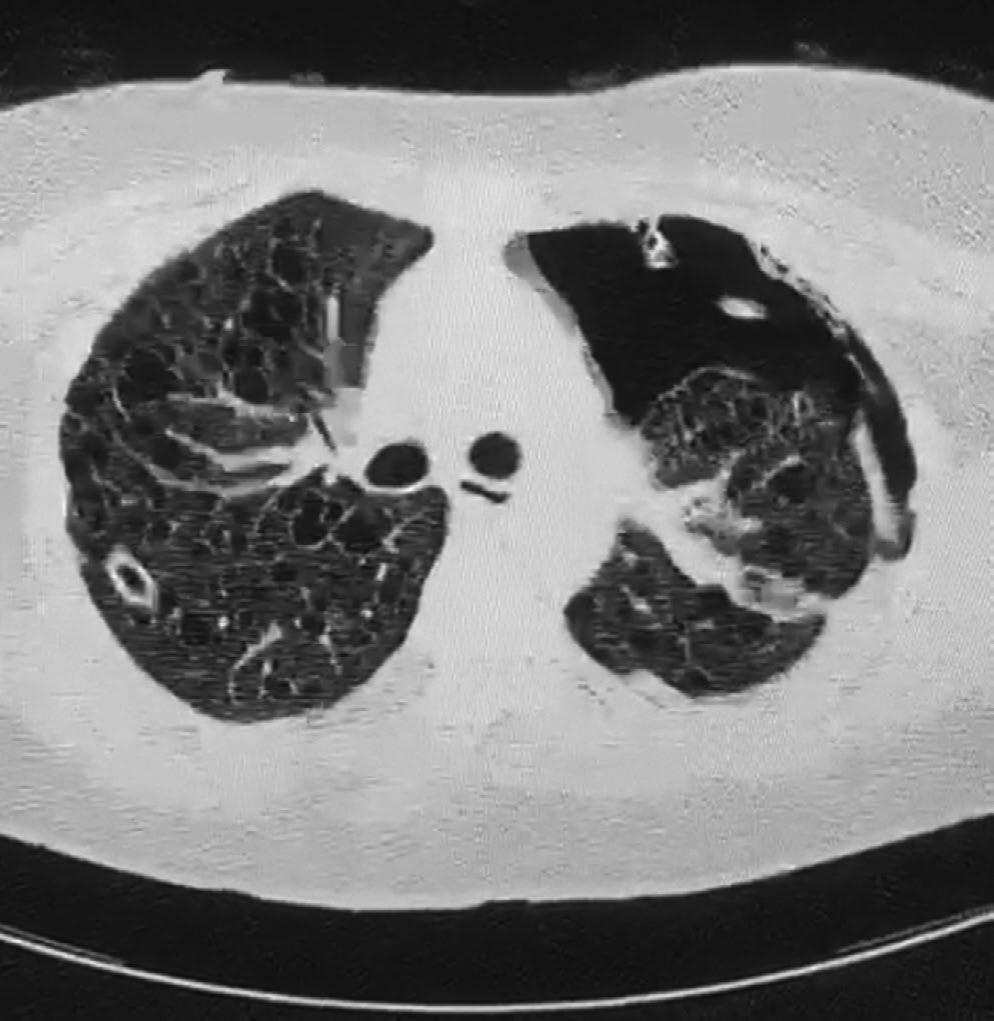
CT scan showing characteristic appearance of LAM
